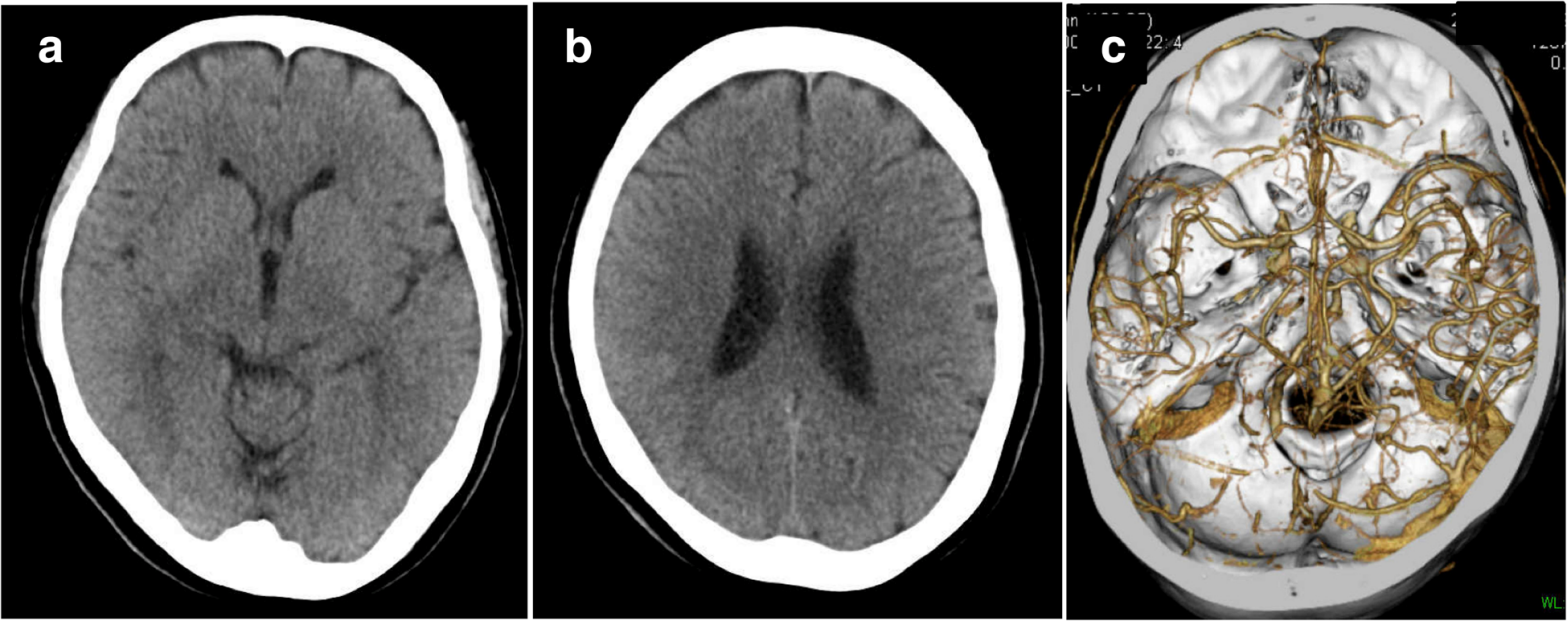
Brain CT images (a, b) do not show a hypodense area at admission, but equivocal findings of effacement in the right temporoparietal region (ASPECT score, 8). CT angiography (c) shows no vascular occlusion or stenosis. CT = computerized tomography, ASPECTS = Alberta Stroke Program Early CT Score
